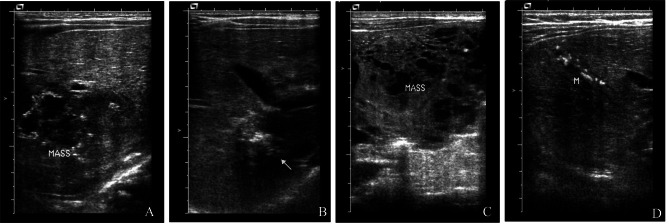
Ultrasound (US) images of the observation (A, B) and treatment groups (C, D) at birth (A, C) and 2 years old (B, D)
